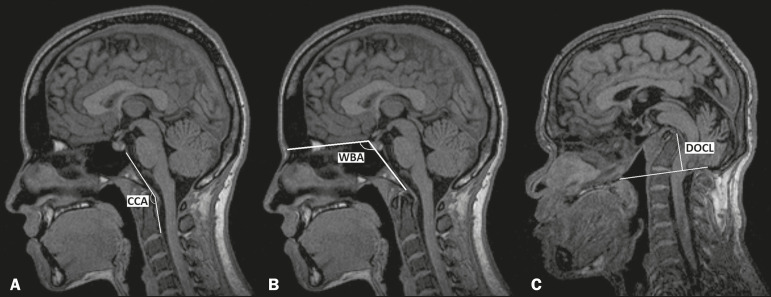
Midsagittal T1-weighted MRI. A: Measurement of the CCA. B: Measurement of the WBA. C: Measurement of the DOCL.
